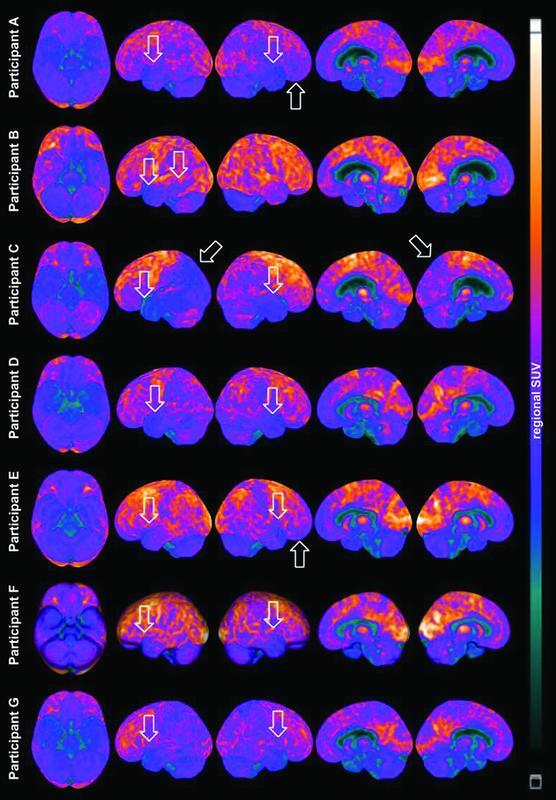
Abbreviation: SUV, Standard Uptake Value.
